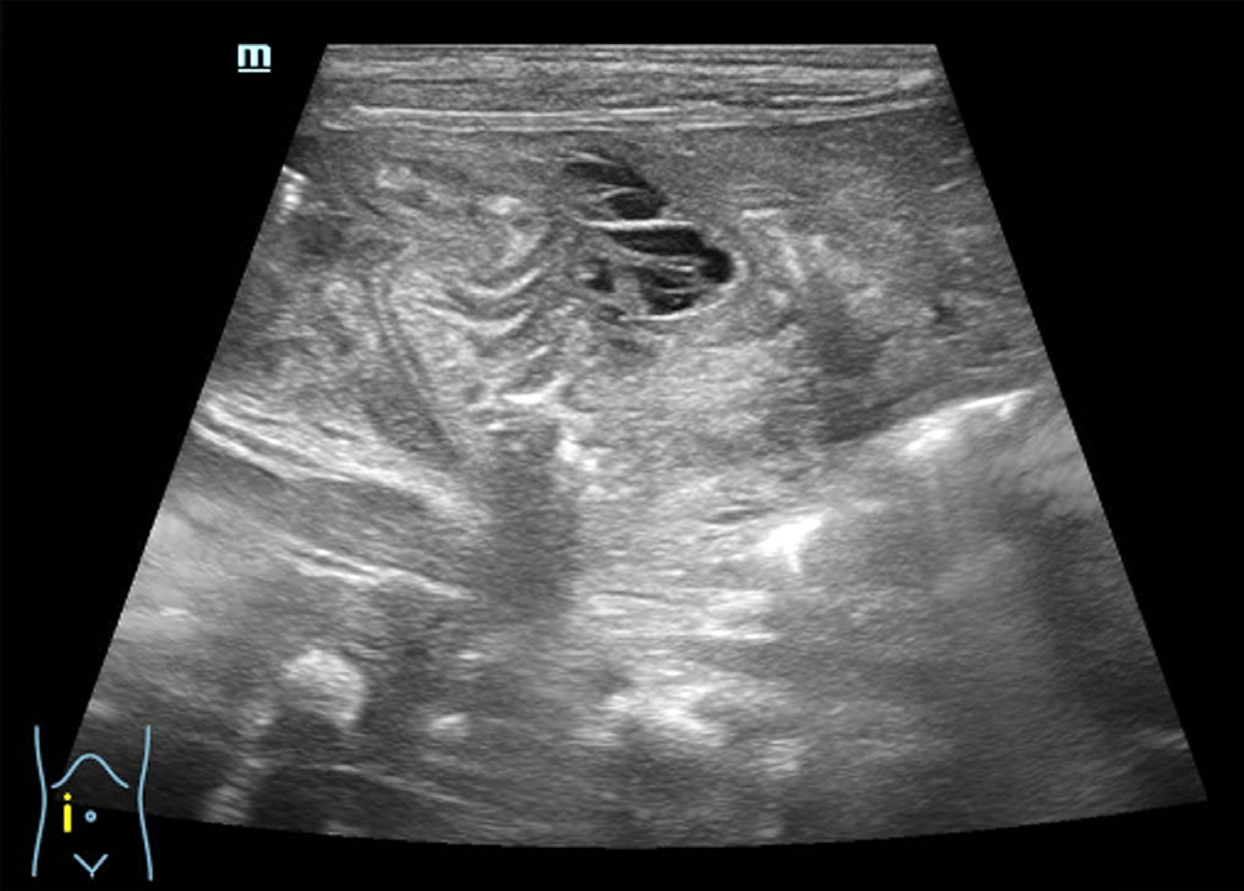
Ultrasonography on third day of admission showing an inflammatory mass
